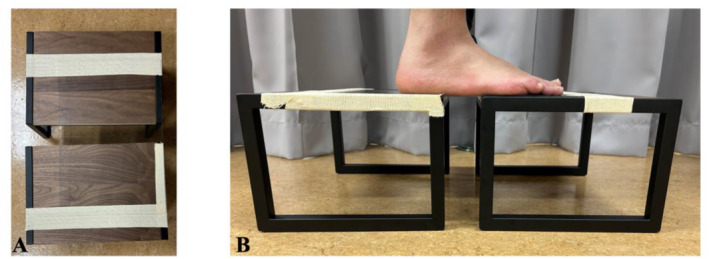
Custom standing platform used for ultrasonographic measurements. (A) PVC plate bridging two 20‐cm‐high platforms with a 6‐cm gap. (B) Side view of the platform setup showing the probe inserted through the gap during the measurement.
